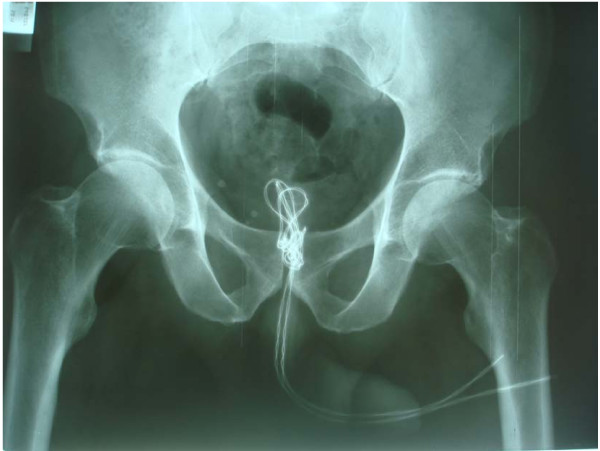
X-ray of kidney, ureter, bladder: coiled up radiopaque wire inside the bladder.
